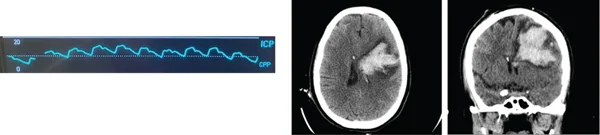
Normal ICP waveform and first head CT scan. Midline shift was 8 mm.
